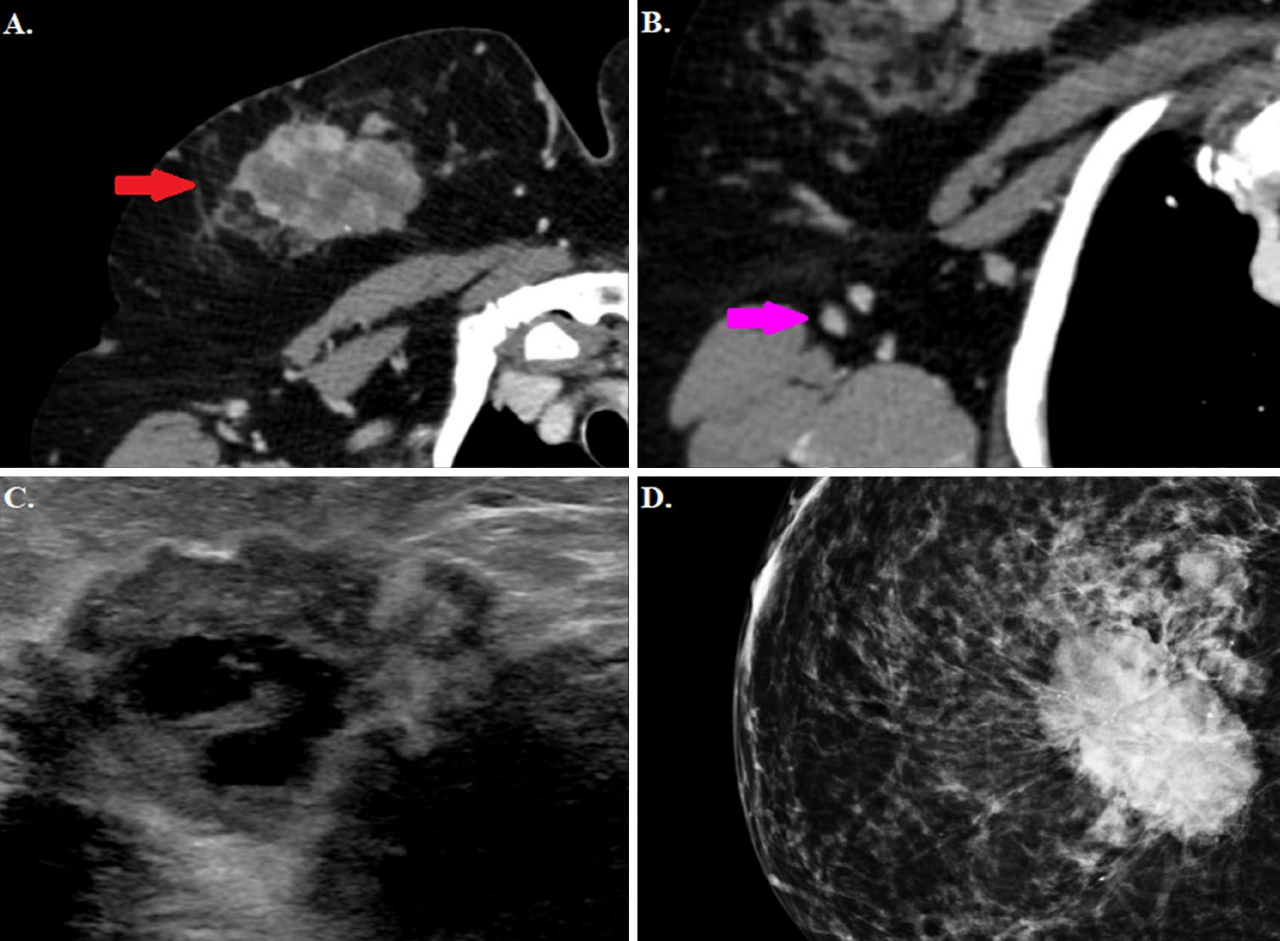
Invasive ductal carcinoma. A Breast tissue CT imaging after intravenous injection of organic iodinated contrast agent showing a nodular tumour with undefined margins and a marked and inhomogeneous contrast enhancement (red arrow); B Homolateral lymph node CT imaging appearing increased in size, with marked contrast enhancement and lacking a clearly recognisable hilum (pink arrow). C Mammary ultrasound with a highly hypoechoic nodule with irregular margins and acoustic shadow. D Mammogram showing a thickened asymmetric breast tissue with irregular margins and intramodular microcalcifications
